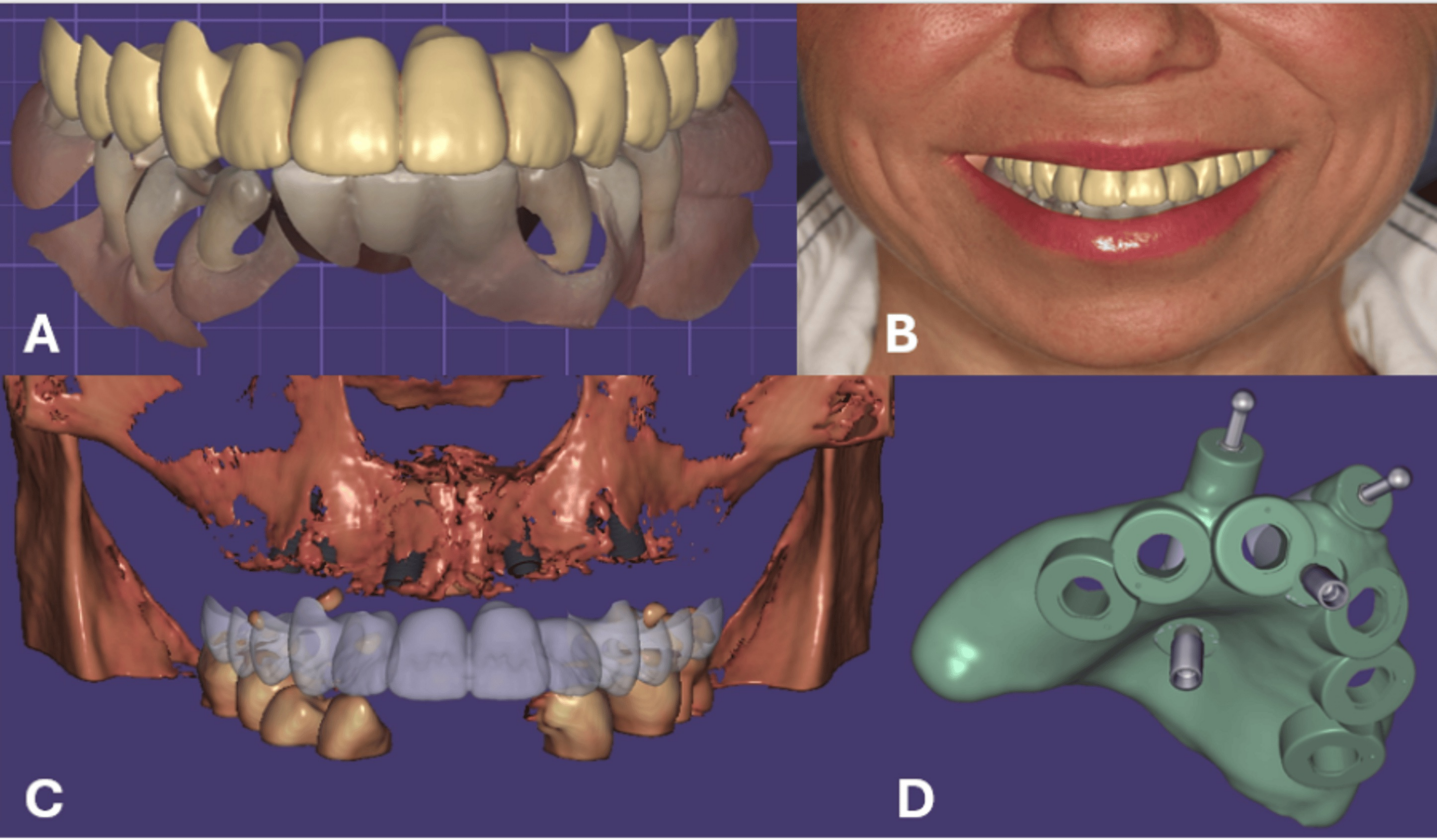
A) Digital patient image was created in “ExoCad” software; B) Digital smile design created after combining information from the extraoral photo. Digital impression derived from intraoral optica and CBCT; C) Virtual position of the implants according to CBCT and DSD; D) Design of the surgical guide.
